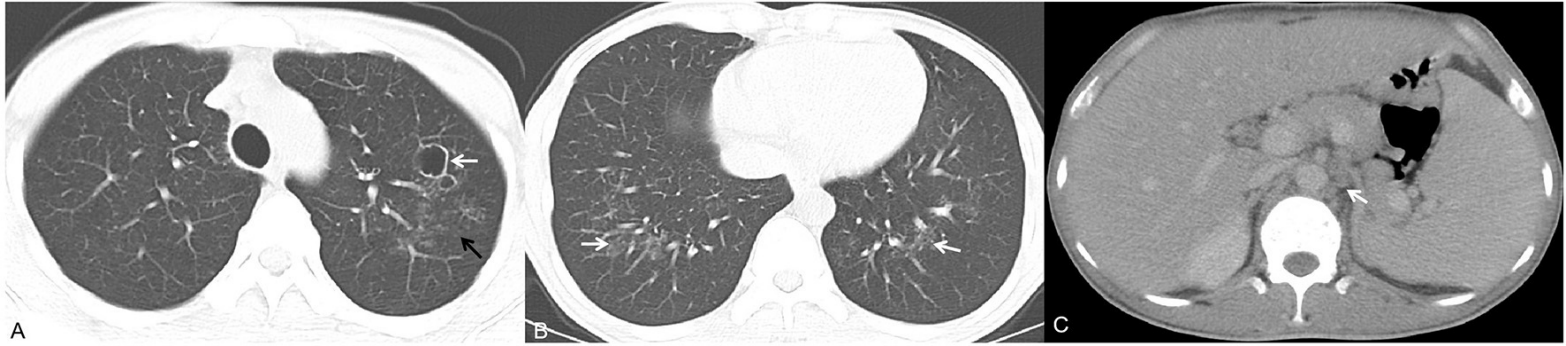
A 40-year-old man presented with fever, cutaneous lesions and ulcers of the oral mucosa. Axial chest CT images (A,B) showed multiple cavities, multiple patches (white arrow) and small nodules (black arrow). Moreover, the patient exhibited homogeneous enhancement of hepatosplenomegaly and enlarged lymph nodes in the retroperitoneum [(C), white arrow].
